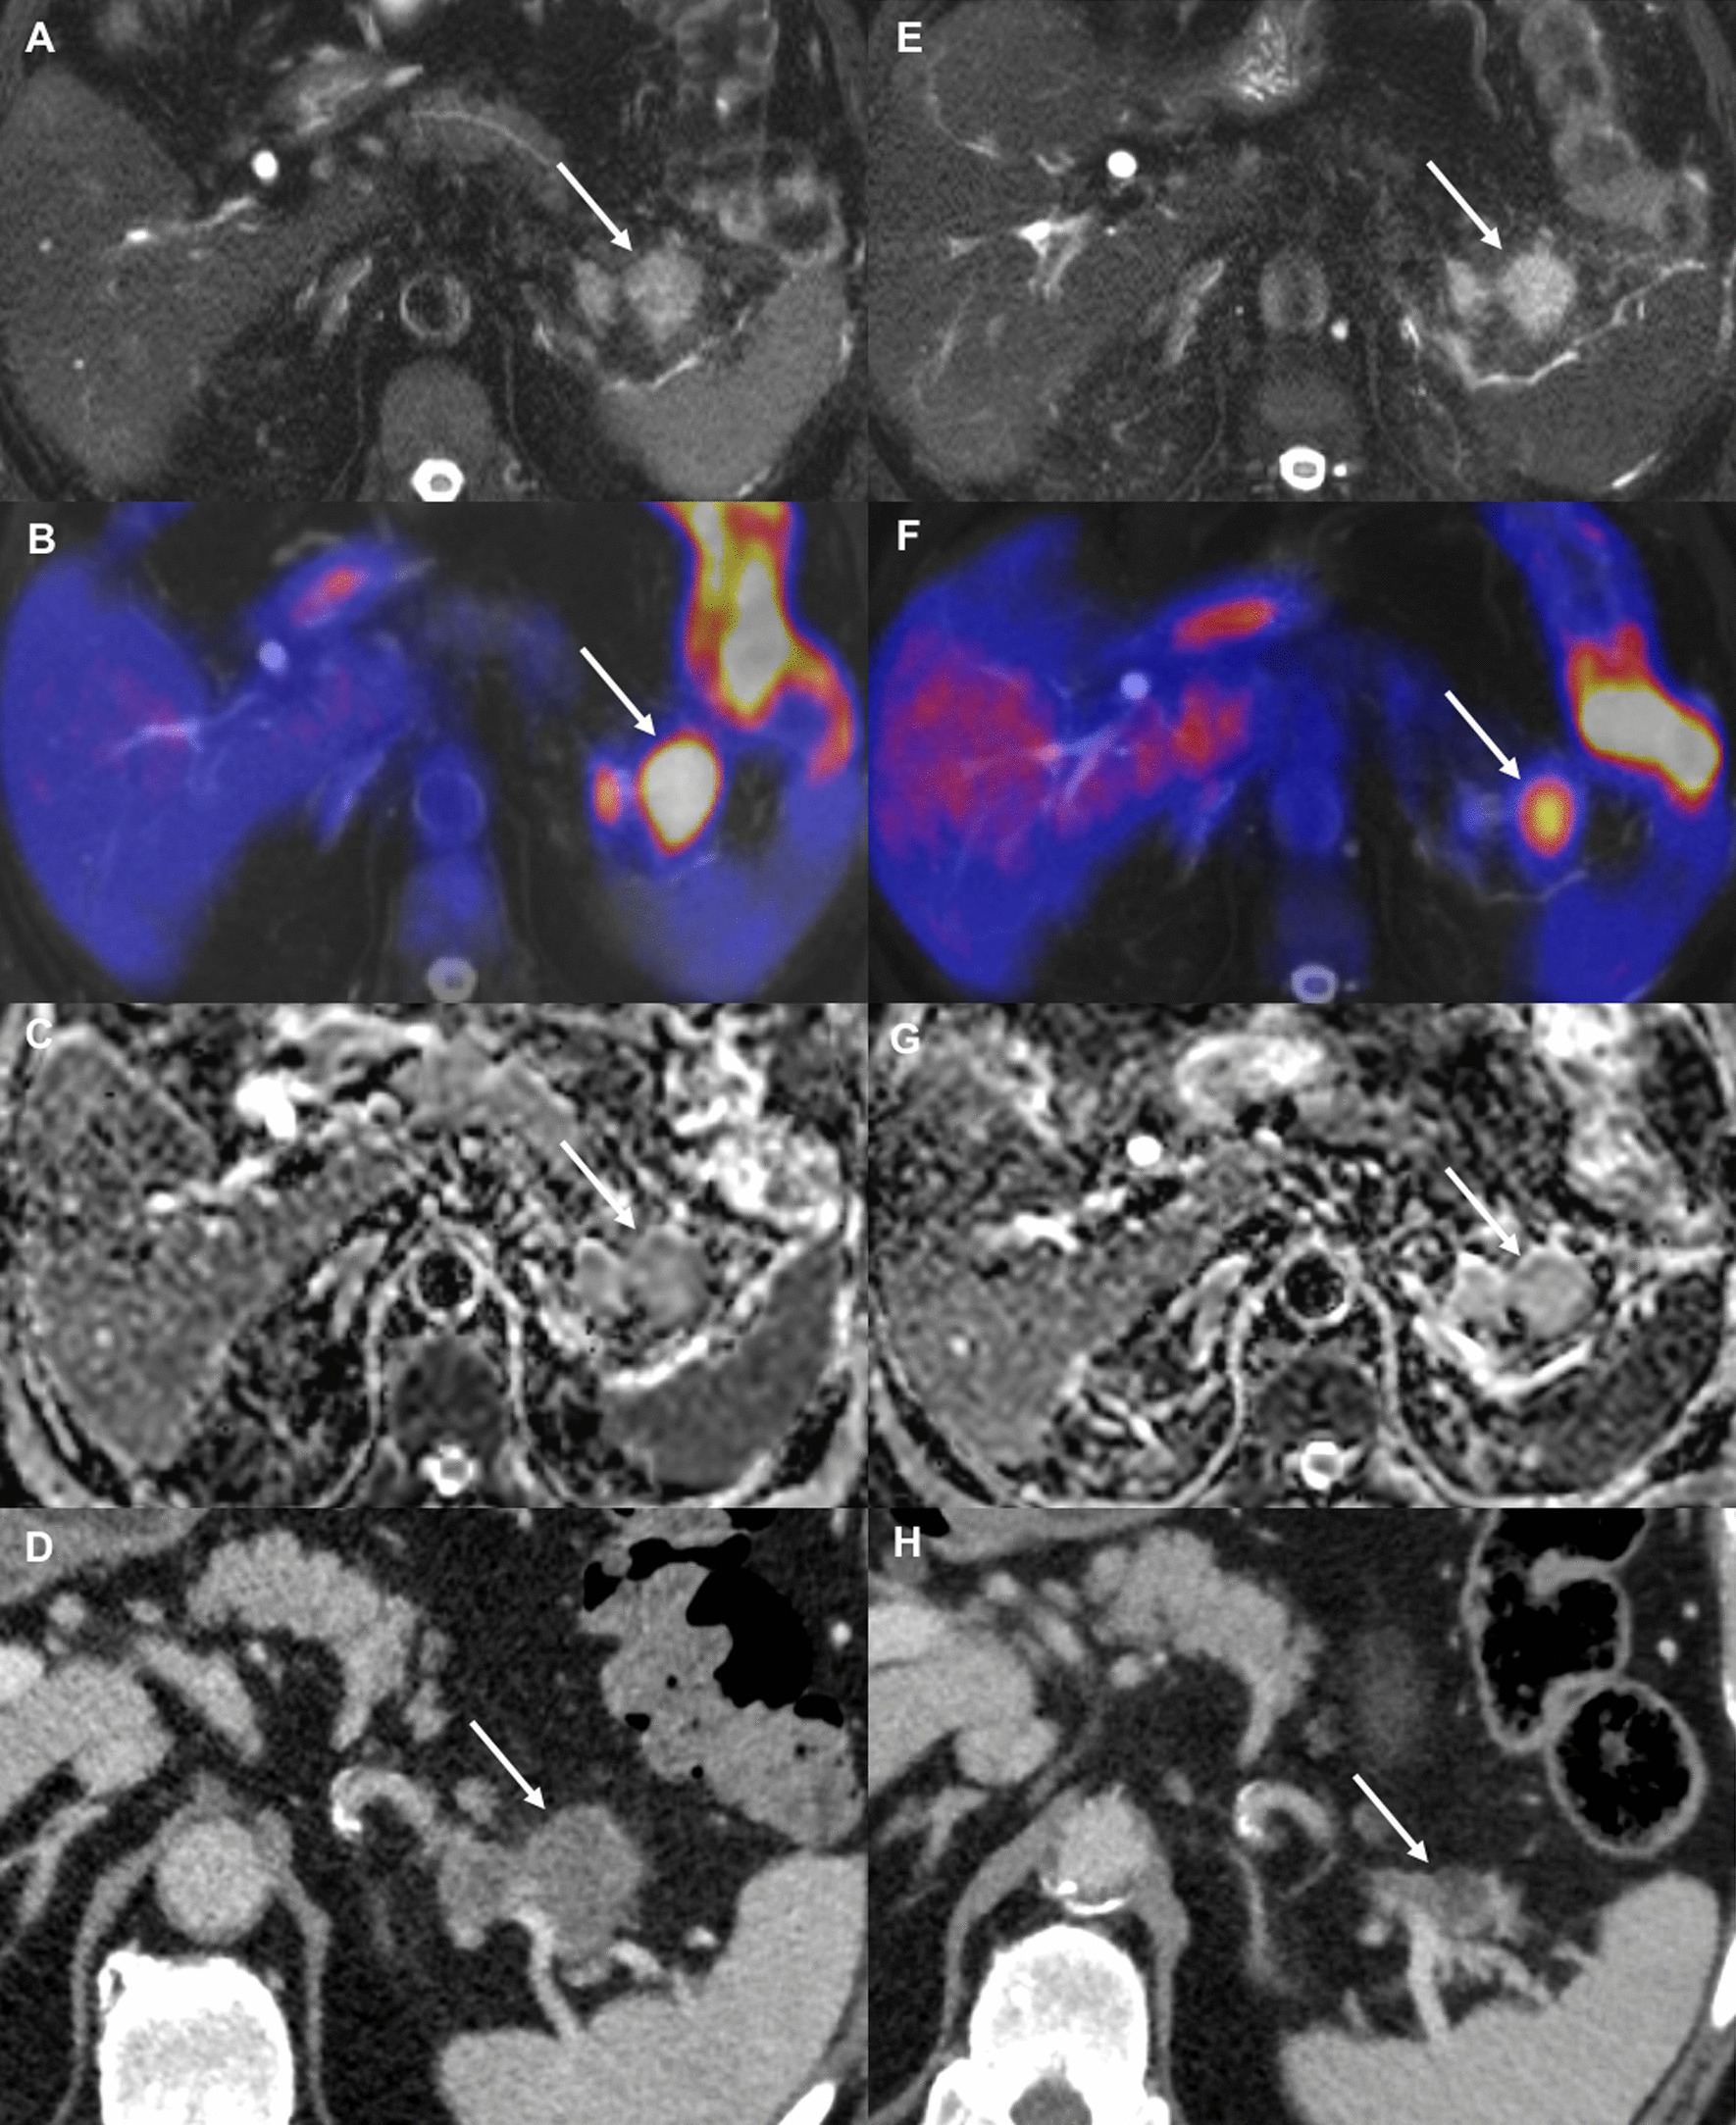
Images from a 69y-old male patient with a PDAC in the pancreatic tale. Images on the left (a–d) were obtained prior to the onset of chemotherapy, images on the right (e–h) were obtained 14 days later, after two cycles of neoadjuvant chemotherapy with nab-Paclitaxel/gemcitabine. Axial T2 FS (a, e) show no change in tumor size. However, PET showed a significant decrease in MTV_50%_ (56.4%) and TLG_50%_ (53.9%) (b, f). Furthermore, a significant increase in ADC_mean_ (44.8%) was seen (c, g). The bottom row displays the baseline CT (d) as well as the follow-up CT (h) after 5 cycles of nab-Paclitaxel/gemcitabine in the same patient. The follow-up CT was obtained after 81 days. Based on RECIST1.1 the patient was stratified as a responder. Left-sided pancreatic resection with splenectomy was performed after the follow-up CT
